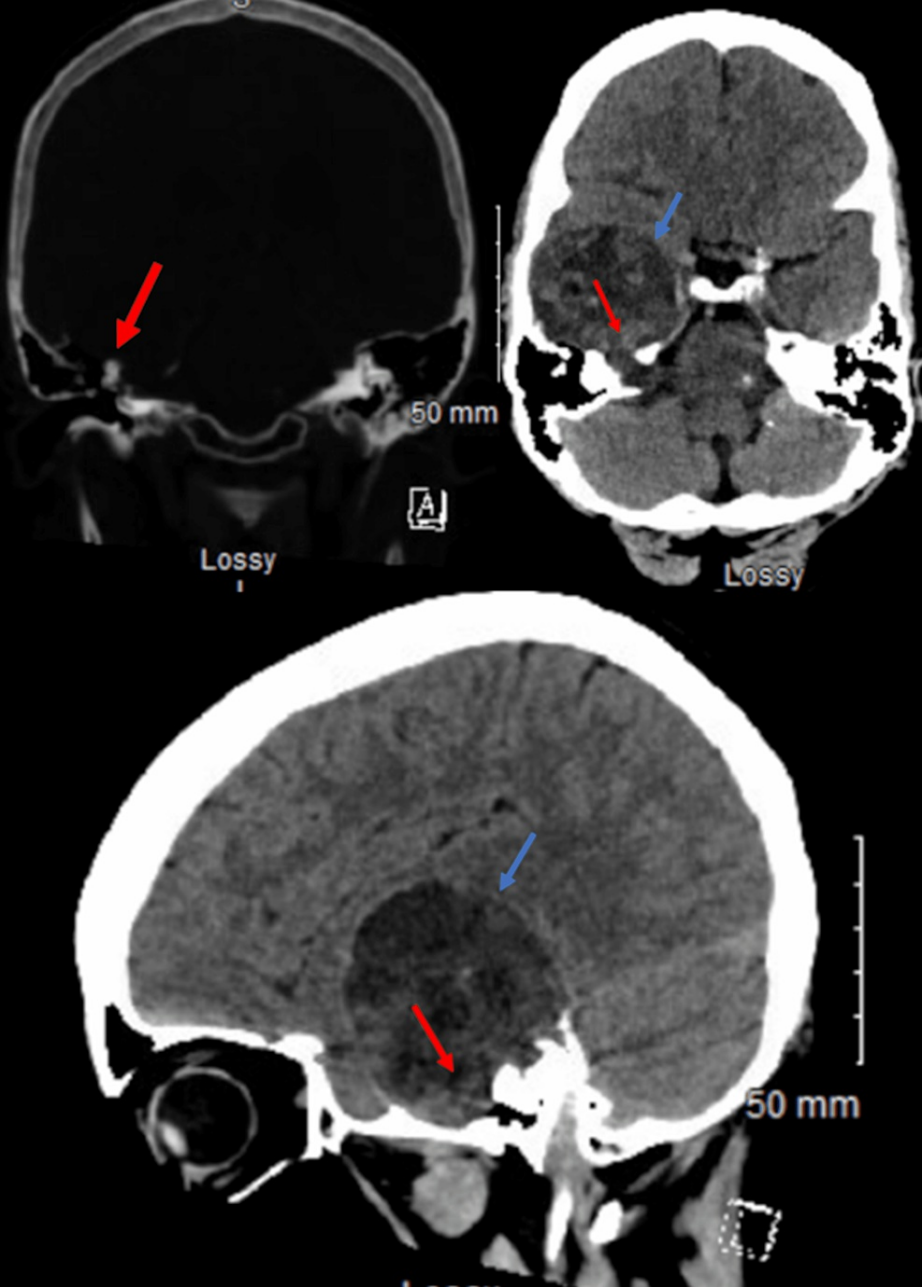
Preoperative CT head: coronal, axial, and sagittal views without contrast The view shows a right 6.2 cm x 5.1 cm x 5.9 cm extra-axial lesion (blue arrow) filling the internal auditory canal and wrapping superiorly and anteriorly across the right temporal bone (red arrow) along the floor of the right middle cranial fossa.
